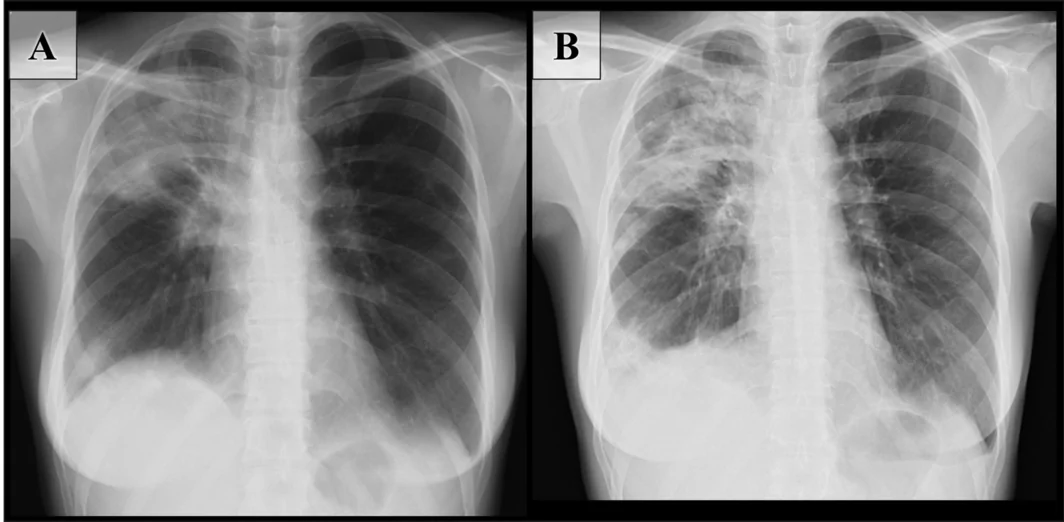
Comparison of chest radiographs. (A) Initial chest radiograph showing a consolidation in the right upper lung field. (B) Chest radiograph showing worsening pneumonia in the right upper lung and pleural effusion.
